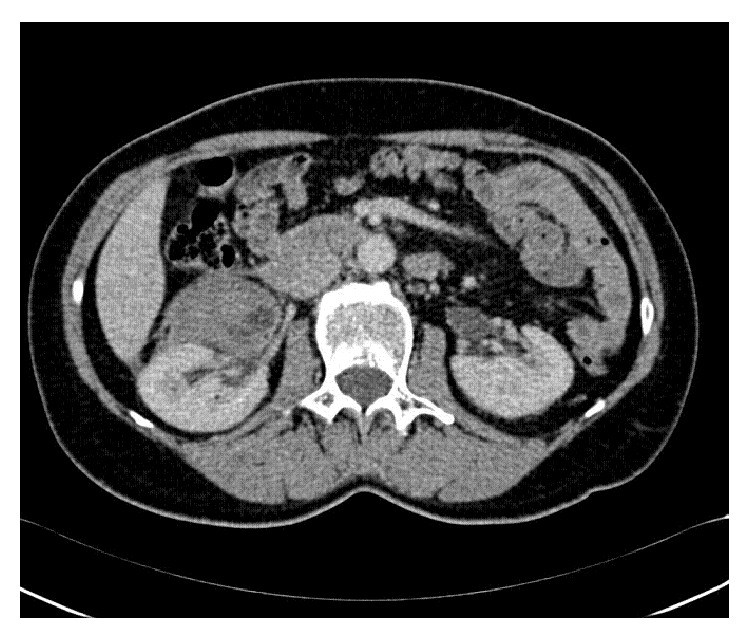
CT scan revealed a right perirenal hematoma after the rupture of the renal angiomyolipoma.
